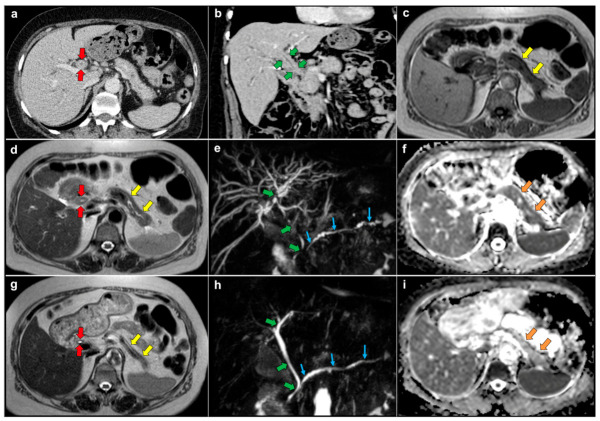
IgG4-related sclerosing cholangitis (IgG4-SC) with concomitant diffuse AIP in a female patient presenting with painless jaundice, elevated bilirubin and increased CA 19-9. (a,b) Axial and coronal portal-phase CT demonstrates marked concentric and symmetrical thickening of the CBD (red arrows), with a long band-like stricture (green arrows) and upstream dilatation of the intrahepatic bile ducts. (c–f) Subsequent MRI, including (c) axial T1WI, (d) axial T2WI, (e) coronal MRCP with MIP reconstruction and (f) ADC map, confirms the circumferential stenosing thickening of the CBD observed on CT (red arrows). Additionally, segmental strictures of the intrahepatic bile ducts with prestenotic dilatation are observed on MRCP (green arrows), consistent with type 2A IgG4-SC according to the cholangiographic classification. Concomitant signs of diffuse AIP are also present, including a “sausage-shaped” pancreas with a hypointense peripancreatic “halo” best visualised on T1WI (yellow arrows), skipped and tapered “icicle-shaped” MPD strictures (blue arrows) and low ADC values (orange arrows). (g–i) Follow-up MRI after two months of steroid therapy, including (g) axial T2WI, (h) coronal MRCP with MIP reconstruction and (i) ADC map, demonstrates resolution of both the concentric thickening and narrowing of the CBD (red arrows) and the segmental strictures of the intrahepatic bile ducts (green arrows), without prestenotic dilatation. Marked improvement of the tapered MPD strictures (blue arrows), decreased pancreatic volume with parenchymal atrophy (yellow arrows) and higher ADC values (orange arrows) are also observed.
